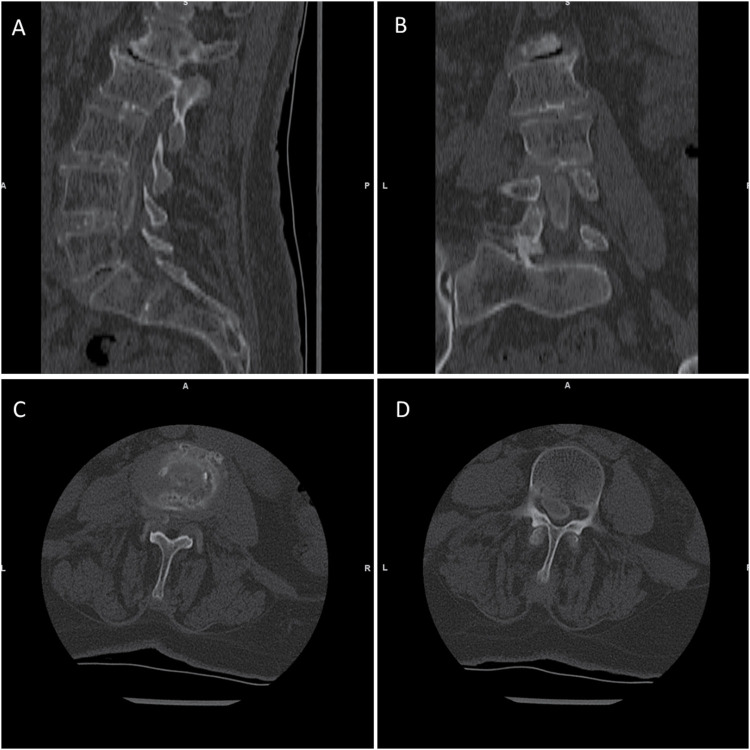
Preoperative Lumbar Spine CT Scan Bone Window. (A) The Sagittal Image Reveals Linear and Punctate Calcifications within the Intervertebral Discs From L2/3 to L4/5, Appearing less Dense than Cortical Bone. Disc Bulging and Herniations are Observed at the Endplates of L2 and L3, as Well as L3/4. Erosive Changes are Noted in the L2/3 Endplates, Accompanied by Moderate Ventral and Dorsal Spondylophyte Formations and Moderate Ventral Spondylophytes on the Superior Endplate of L4. In the Anterior Spinal Canal, Elongated, Spindle-Shaped to Lobulated, Inhomogeneous Calcifications Extend Between L2 and L5, With Resulting Severe Spinal Stenosis at L3/4 and L4/5 (B) the Coronal Image Demonstrates the Calcified Intraspinal Mass Predominantly Located on the Left Side, with its Widest Transverse Diameter at the Level of the L4 Vertebral Body. (C) Axial Imaging at the L3-L4 Intervertebral Disc Shows Well-Defined Linear, and Punctate Calcifications, Less Dense than Cortical Bone. (D) The Axial Image at the L4 Vertebral Body Reveals the Presence of the Calcified Intraspinal Mass, Measuring Approximately 18 mm × 11 mm in its Largest Axial Diameter, Occupying the Anterior Spinal Canal and Most Likely Associated with the Posterior Longitudinal Ligament.
